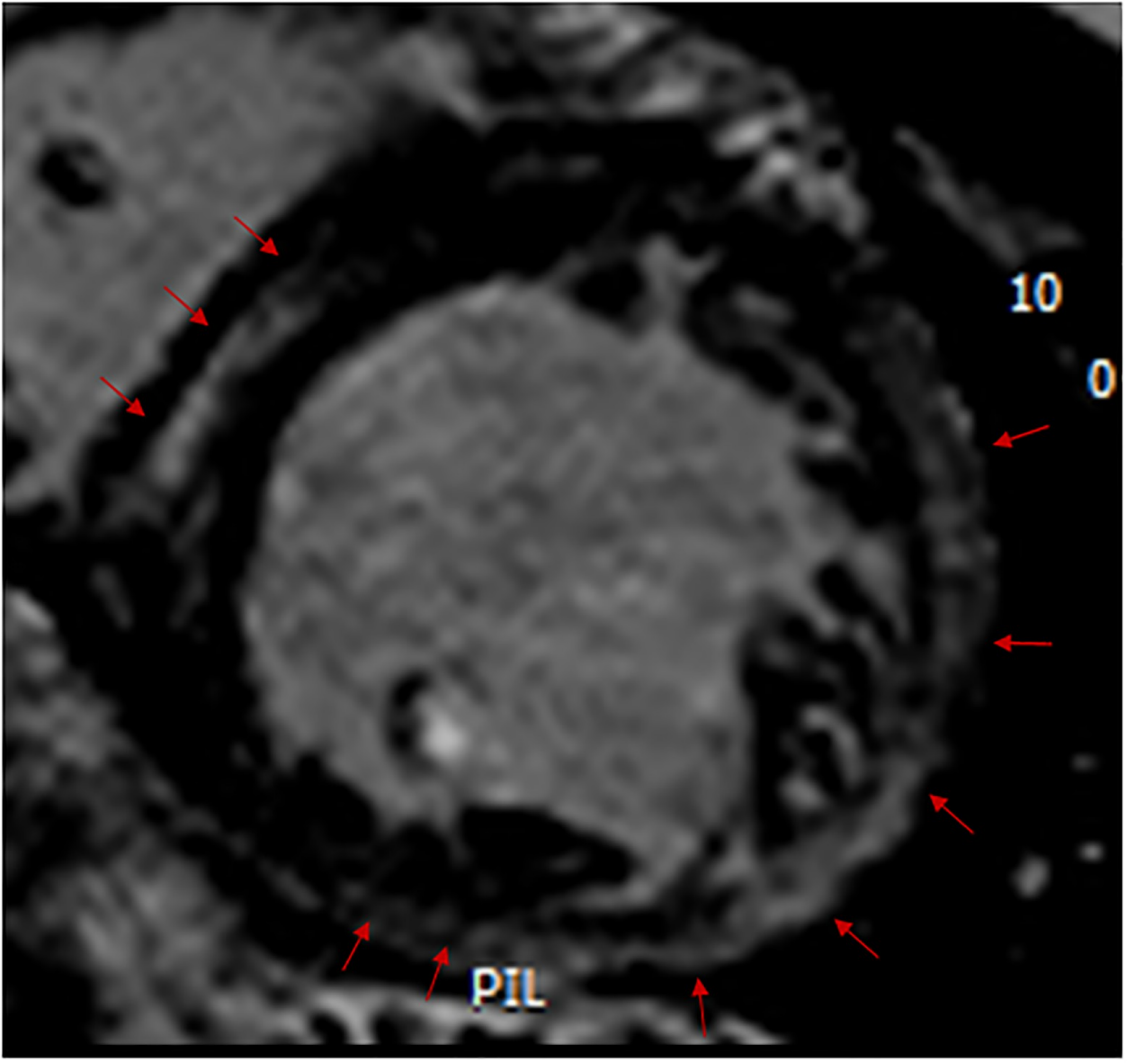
A cardiac magnetic resonance image showing a short-axis section of the left ventricle, with the red arrows on the left showing mid myocardial late gadolinium enhancement in the septal wall and the red arrows to the right of and underneath the ventricle showing epicardial late gadolinium enhancement in the anterolateral and inferolateral walls.
